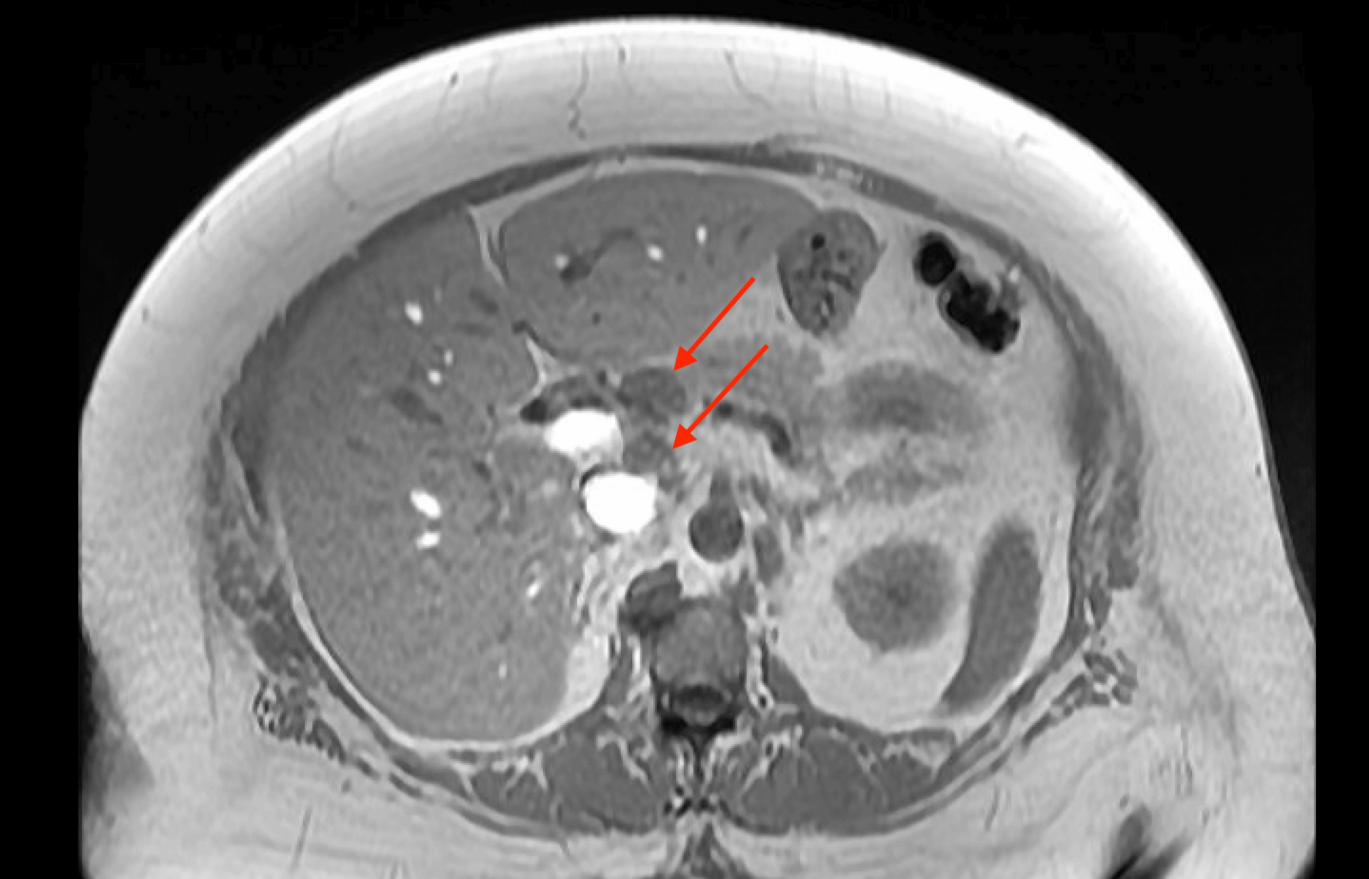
T1-weighted MRI showing peripancreatic lymphadenopathy.
